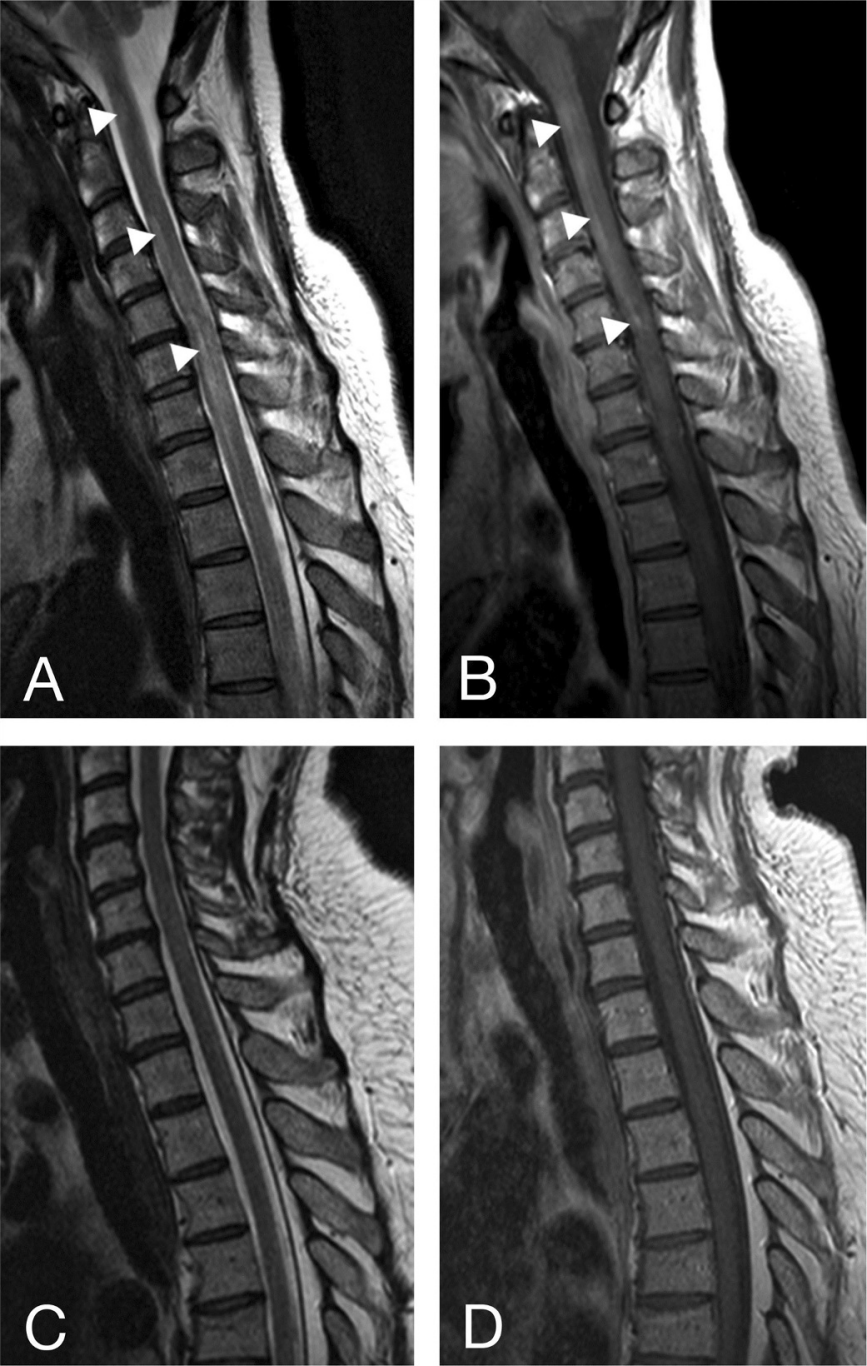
Sagittal T2-weighted (A) and T1-weighted, gadolinium enhanced (B) MRI pictures: Confluent contrast-enhanced lesions in the cervical spinal cord extending to the caudal medulla oblongata (A and B, white arrows); no evidence of myelitis after cyclophosphamide therapy (C and D).
